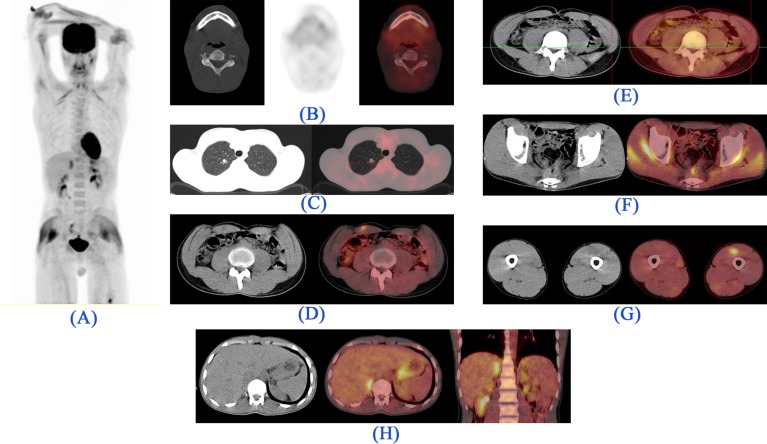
^18^FDG-PET/CT images. *(A). Maximum intensity projection (MIP): *Foci of metabolically active lesions are visualized superior to the right kidney, right mid abdominal region, and the posterior pelvic cavity. In addition, diffuse metabolic activity is noted in skeletal muscles owing to physical activity in the morning of the study. Of note, due to technical issues the imaging of the lower extremities was performed separately. *(B). Trans-axial CT (left), PET (middle) and Fused (right) images:* Comparing with the previous study, the mandibular bone lesion is healed without evidence of remaining tumoral lesion. *(C).*
